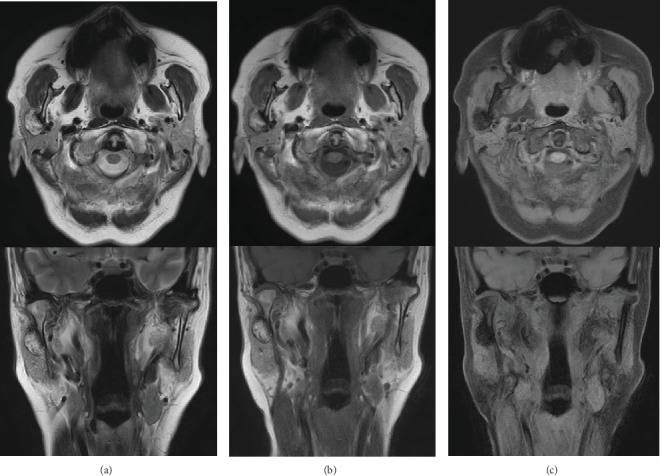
(a) Top: axial section of the magnetic resonance imaging (MRI) scan on T1. Bottom: coronal section of the MRI scan on T1. (b) Top: axial section of the MRI scan on T2. Bottom: coronal section of the MRI scan on T2. (c) Top: axial section of the MRI scan on T1 with fat suppression. Bottom: coronal section of the MRI scan on T1 with fat suppression.
